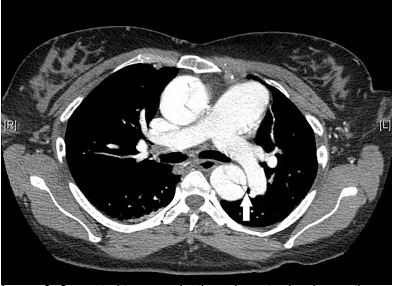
Computed tomography thoracic aorta showing aortic dissection (arrow).
